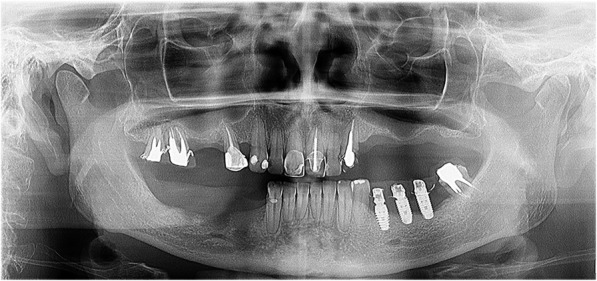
Panoramic radiograph at 7-month follow-up
